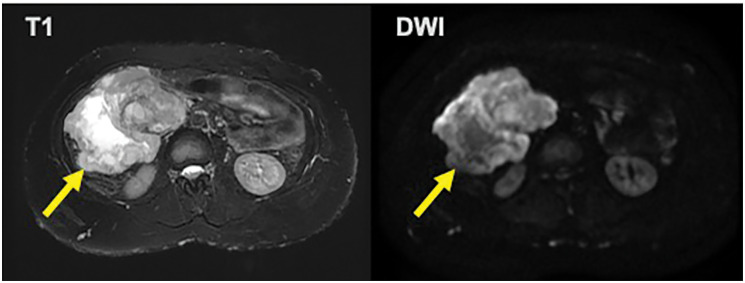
The tumor (arrow) showed high signal intensity on T2 and diffusion-weighted magnetic resonance imaging.
